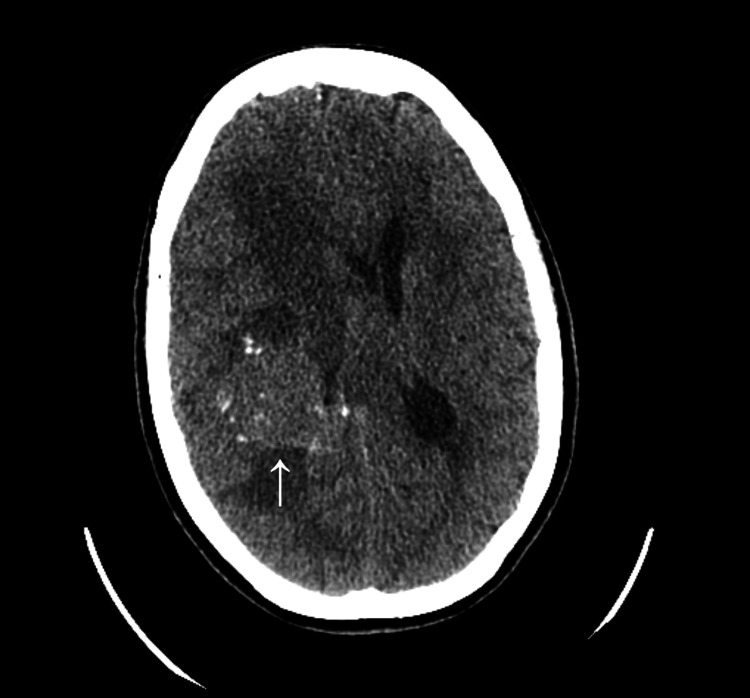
CT scan of the head without contrast showing a hyperattenuating, partially calcified mass (white arrow) measuring approximately 3.7 × 3.6 cm, with surrounding vasogenic edema involving the frontotemporal region.
